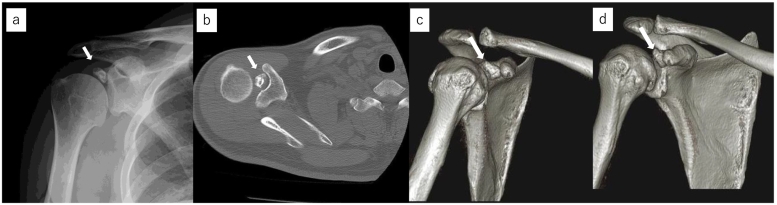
A large loose body was observed within the glenohumeral joint on the plain radiograph and CT images. The loose body is indicated by an arrow. a: The plain radiograph. b: Axial view of plain CT. c: 3D CT imaging revealed the presence of a loose body around the coracoid process. d: Bone spur formation was observed at the anterior edge of the glenoid cavity of the scapula.
